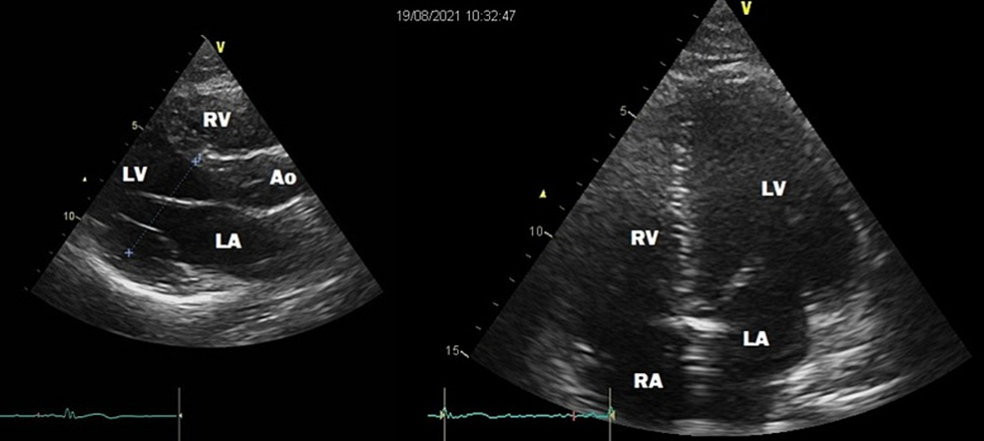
Parasternal long axis and apical four-chamber views of the heart in the left panel and right panel, respectively. Pericardial effusion was disappeared. LV: left ventricle, RV: right ventricle, LA: left atrium, RA: right atrium, Ao: Aorta
